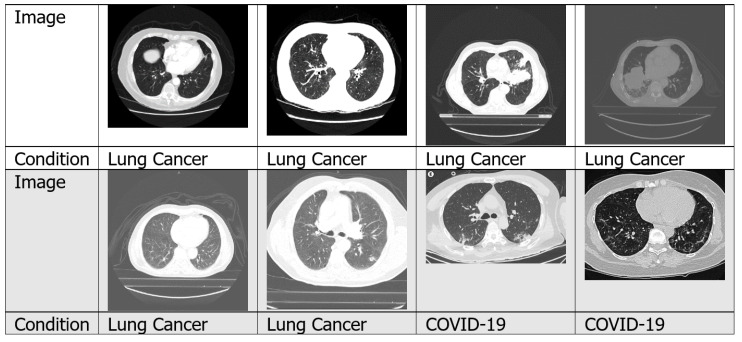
Examples of CT scan images.
